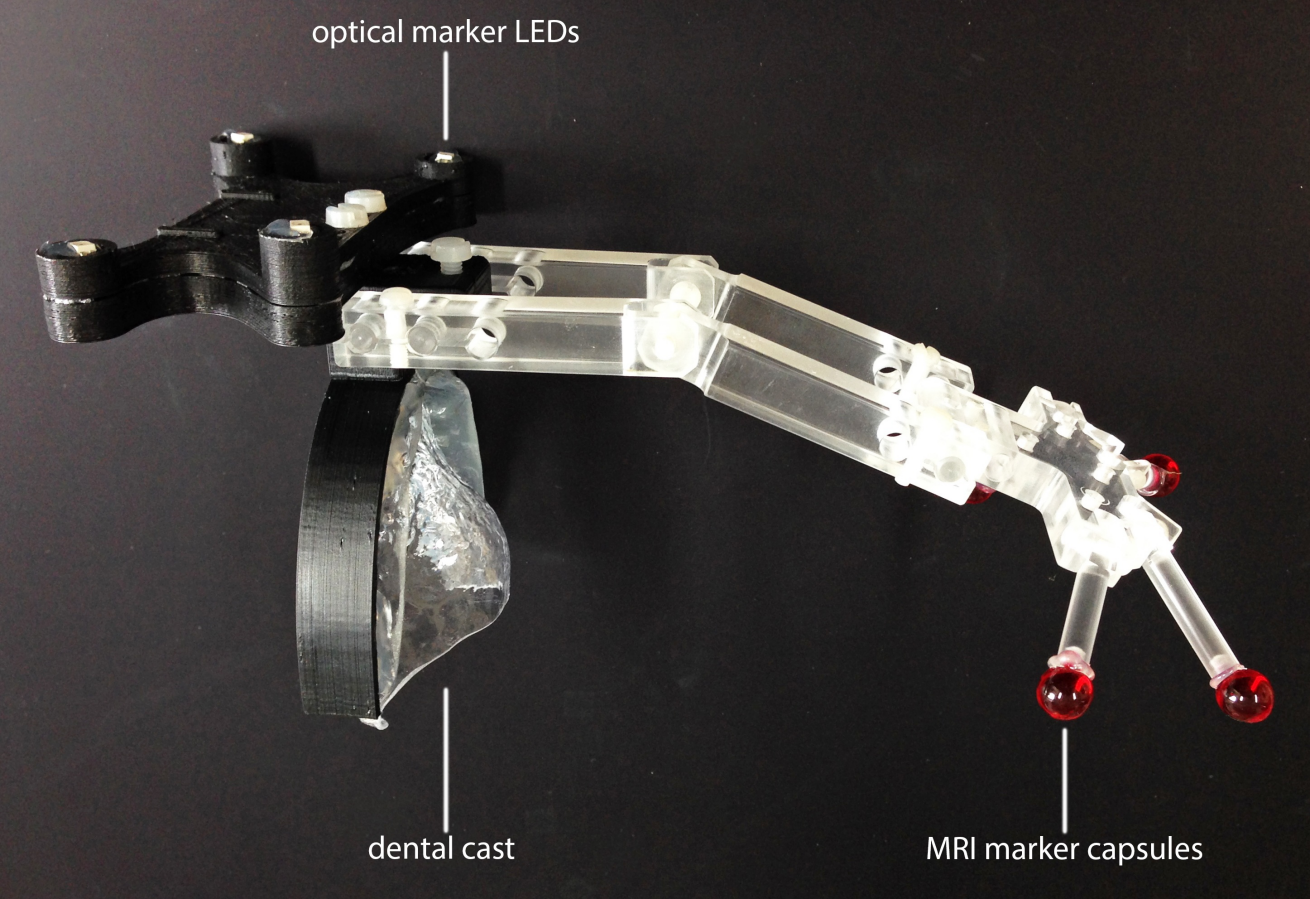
Marker geometry utilized for the matching of MRI and NIR scans
